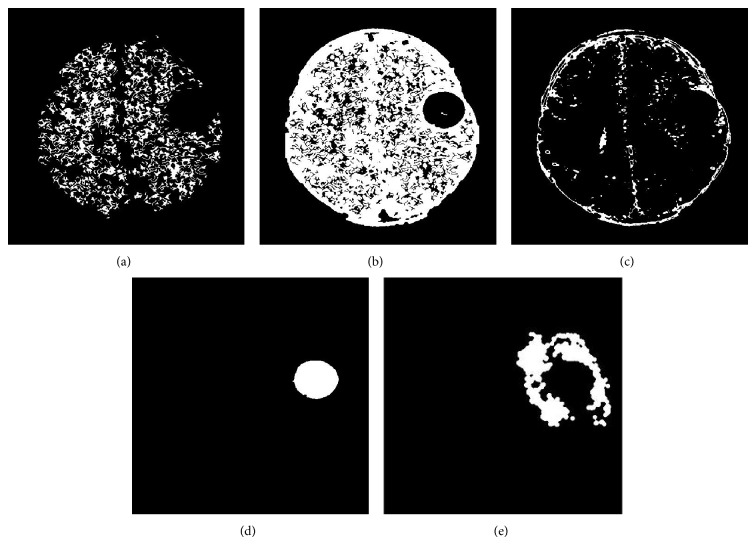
Segmented MRI brain tissues: (a) WM, (b) GM, (c) CSF, (d) tumor, and (e) edema.
